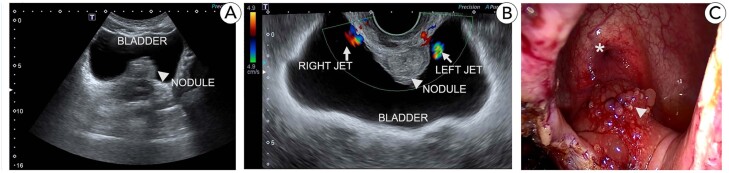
Nodule (arrowhead) identified at transabdominal US (A). Transvaginal US (B) was able to demonstrate bilateral urethral patency using doppler (arrows) despite the proximity to the nodule (arrowhead). Laparoscopic view (C) of the nodule (arrowhead) and bladder trigone (asterisk) just before partial cystectomy.
